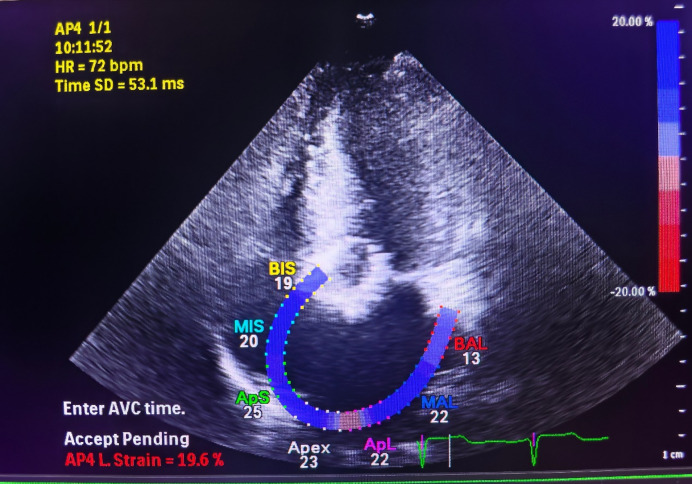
Speckle-tracking echocardiography for left atrial strain analysis.
